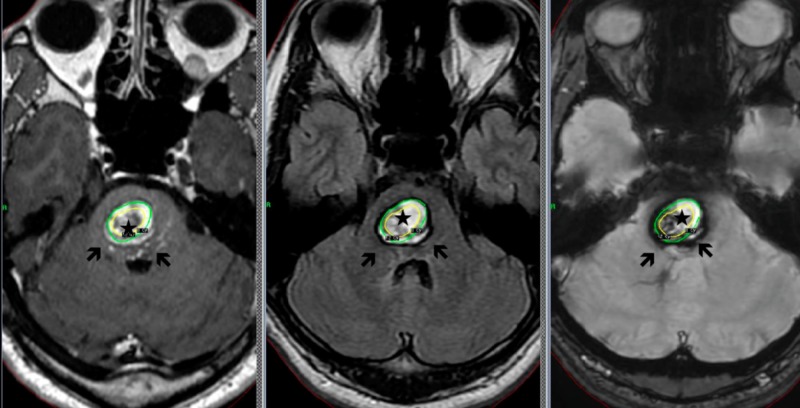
Cavernous malformation in the pons Only the core of cavernous malformation encircled with a yellow line (*) was irradiated, and the surrounding layer with hematomas (arrows) were out of target. Left: T1-weighted image; center: T2-weighted image; right: FLAIR (Fluid-attenuated inversion recovery) image
